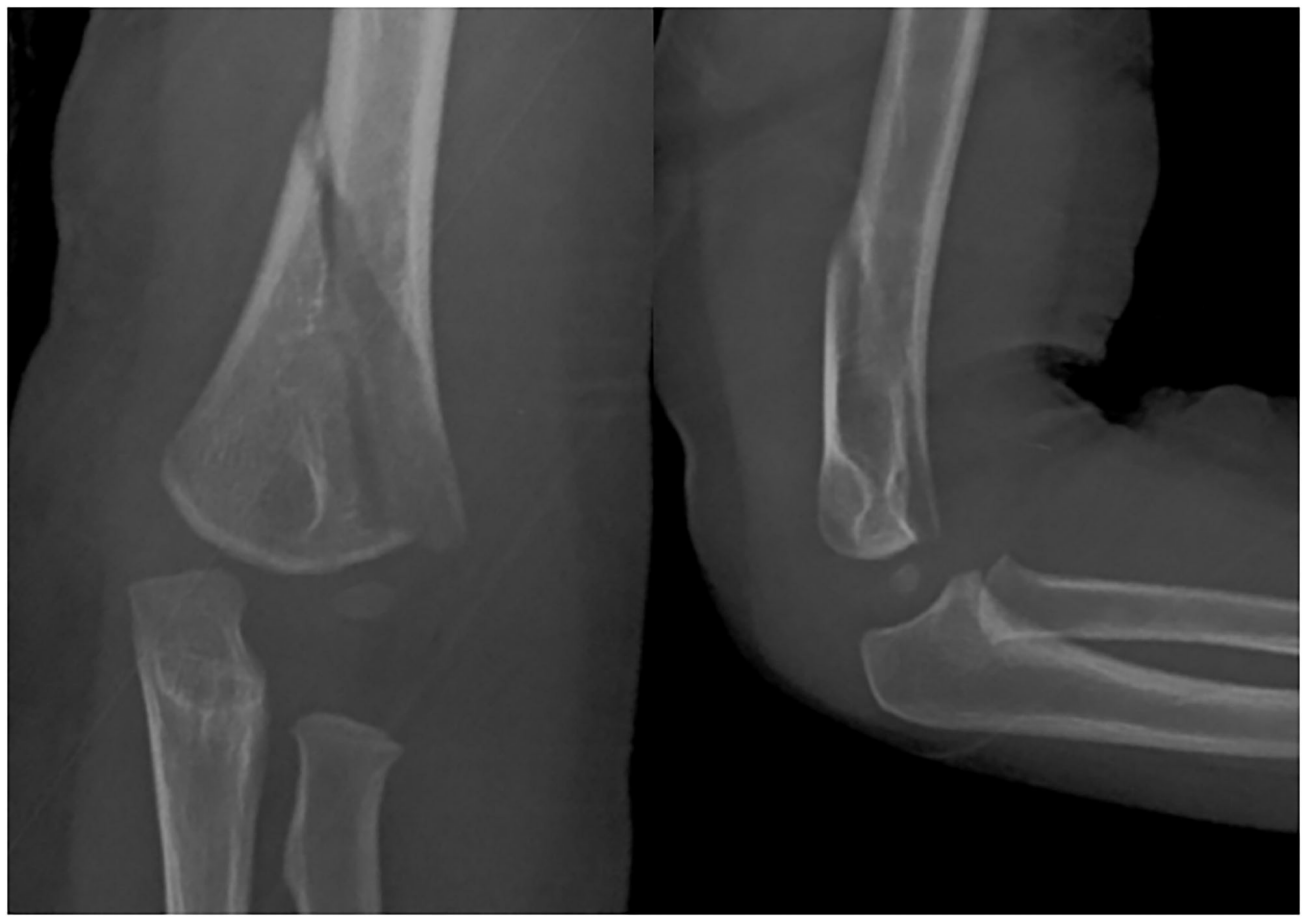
A 2-year-old child fell from the bed and had a direct contact injury to the left elbow. The radiographs demonstrate a completely displaced medial high-long oblique supracondylar humeral fracture.
